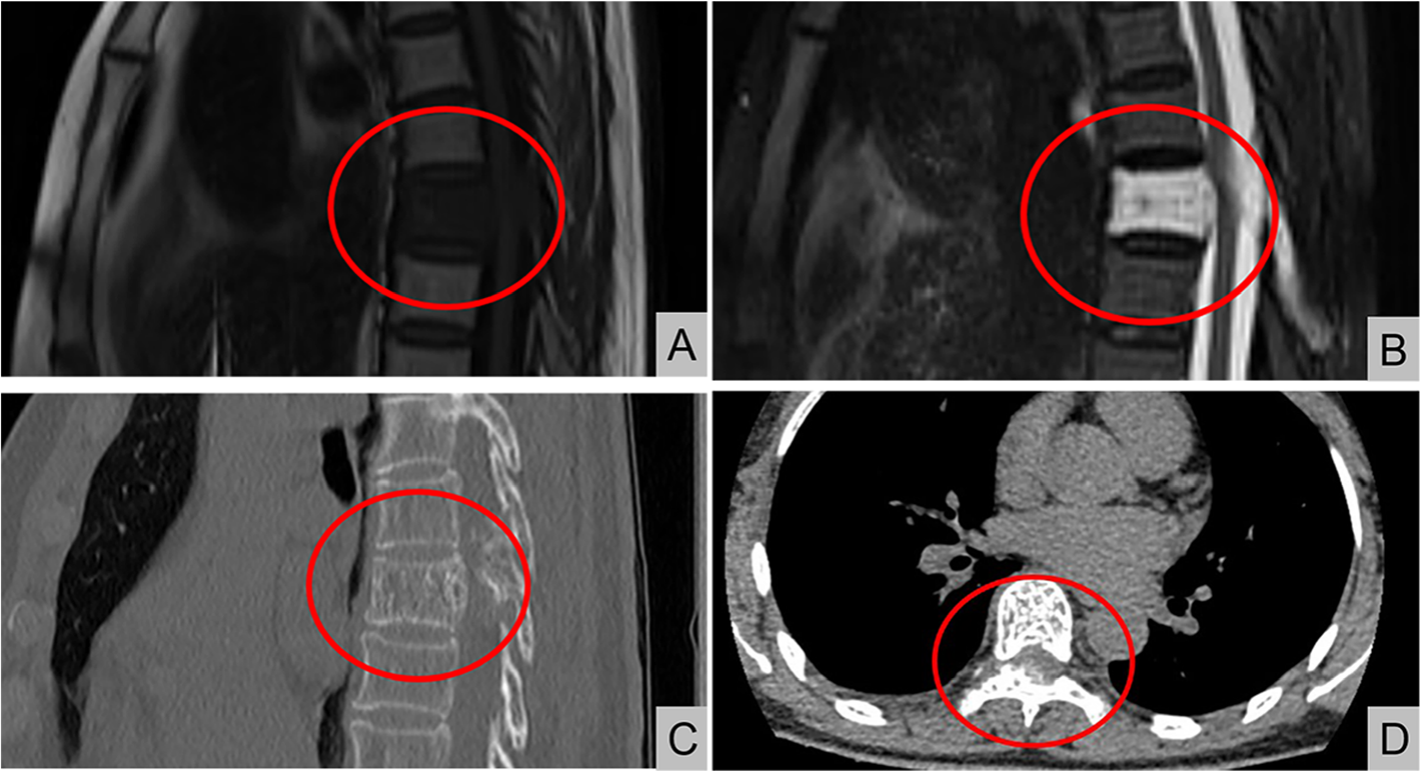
A 69 year-old female patient who had a T7 hemangiomas (typical MRI and CT scans)
